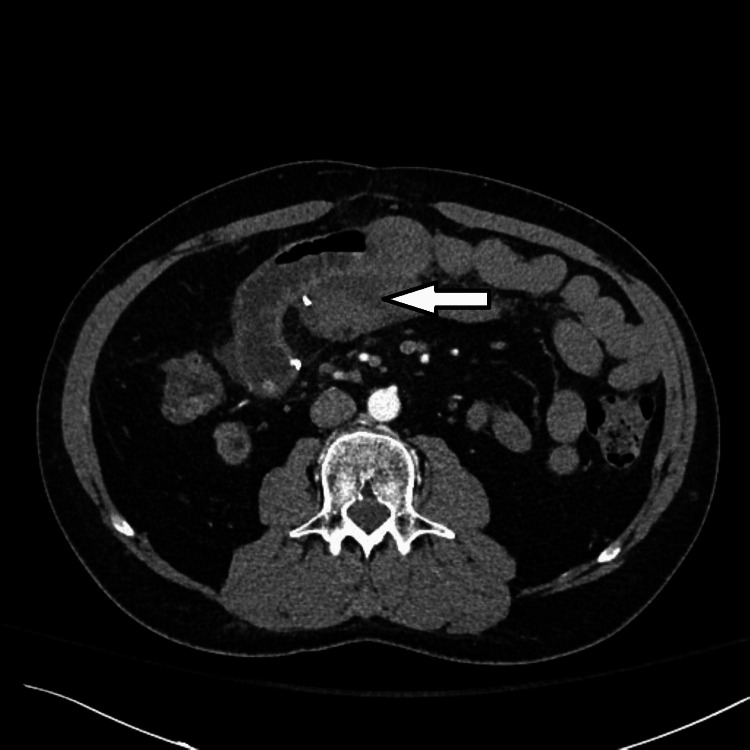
Transverse section of contrast-enhanced abdominal CT scan showing hypoenhancing pancreatic graft CT: computed tomography
